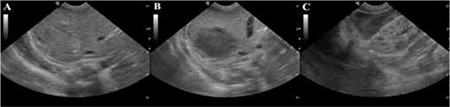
A) Hematoma located on posterior segment of the right liver lobe. B) Hypoechoic cystic lesion located on posterior segment of the right liver lobe on axial plane. C) Control ultrasonography revealed hypoechoic hematoma in the subhepatic region.
